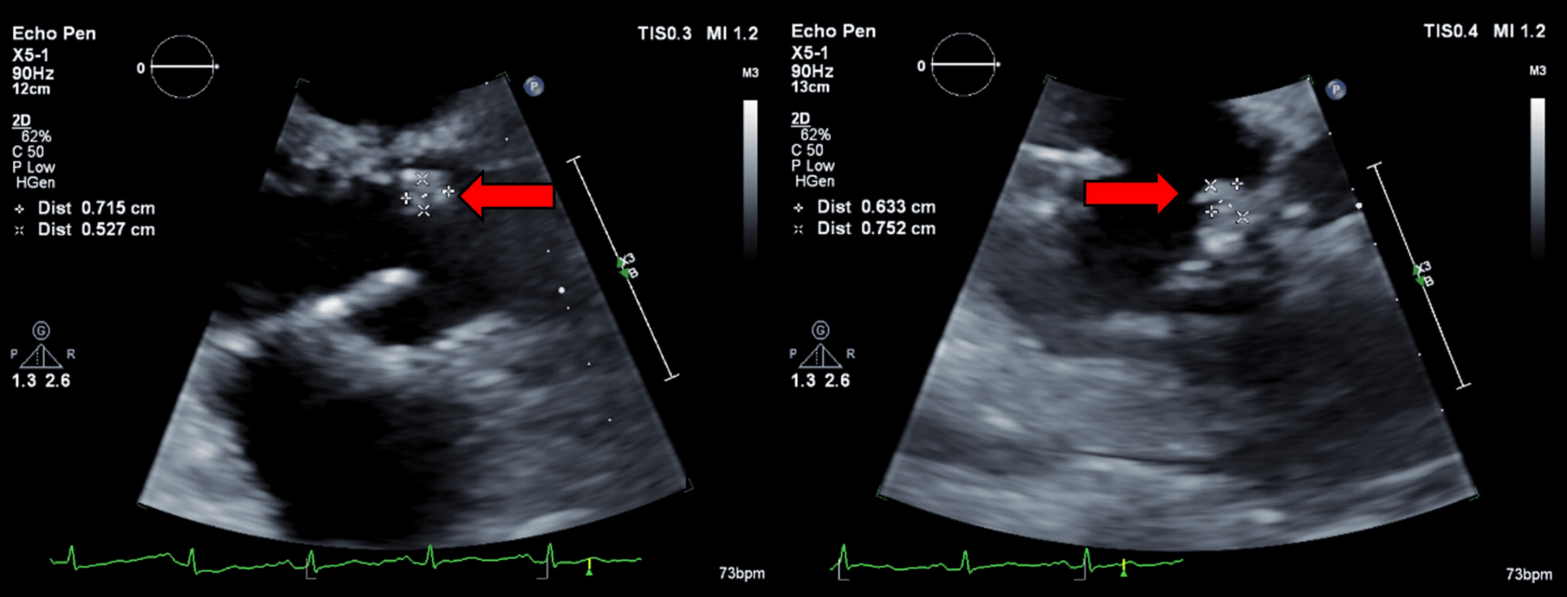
Repeat TTE redemonstrating aortic valve vegetation (red arrows) with measurements. TTE = transthoracic echocardiogram
